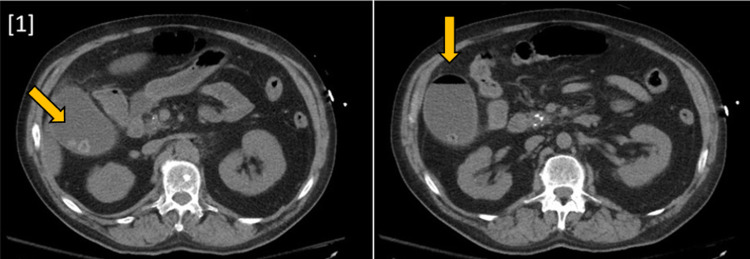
CT abdomen and pelvis without contrast showing a distended gallbladder with air-fluid collection and multiple stones as well as a peri-cholecystic fluid reaction.
